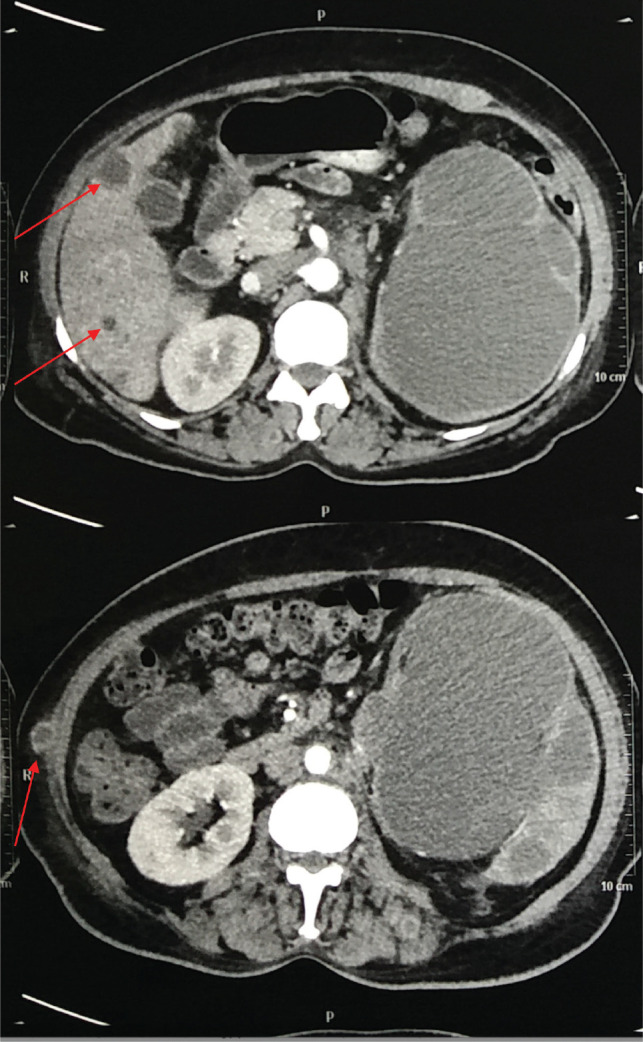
Contrast-enhanced CT scan of the abdomen and pelvis (Transverse section) representing liver and skin metastasis (red arrow).
